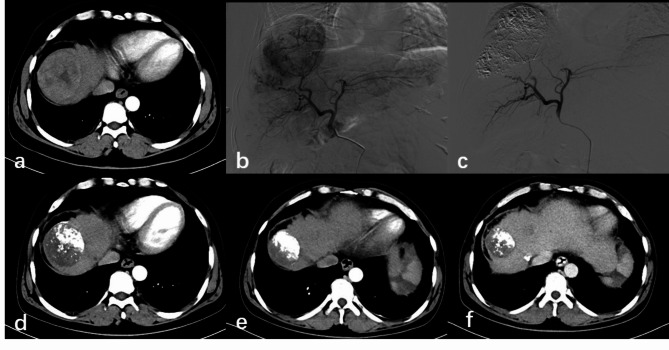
A 50-year-old male patient with hepatocellular carcinoma received DEB-TACE combined with CSMs and 5-Fluorouracil. (a) Preoperative enhanced CT revealed lesions located in the dome of the right hepatic lobe with uneven enhancement. (b-c) Intraoperative DSA angiography showed rich tumor vasculature, which significantly decreased after embolization with CSMs mixed with iodized oil. (d-f) Follow-up enhanced CT at 1, 3, and 6 months postoperatively revealed iodized oil deposition within the tumor, with gradual reduction in volume and absence of obvious enhancing components, suggesting complete remission.
